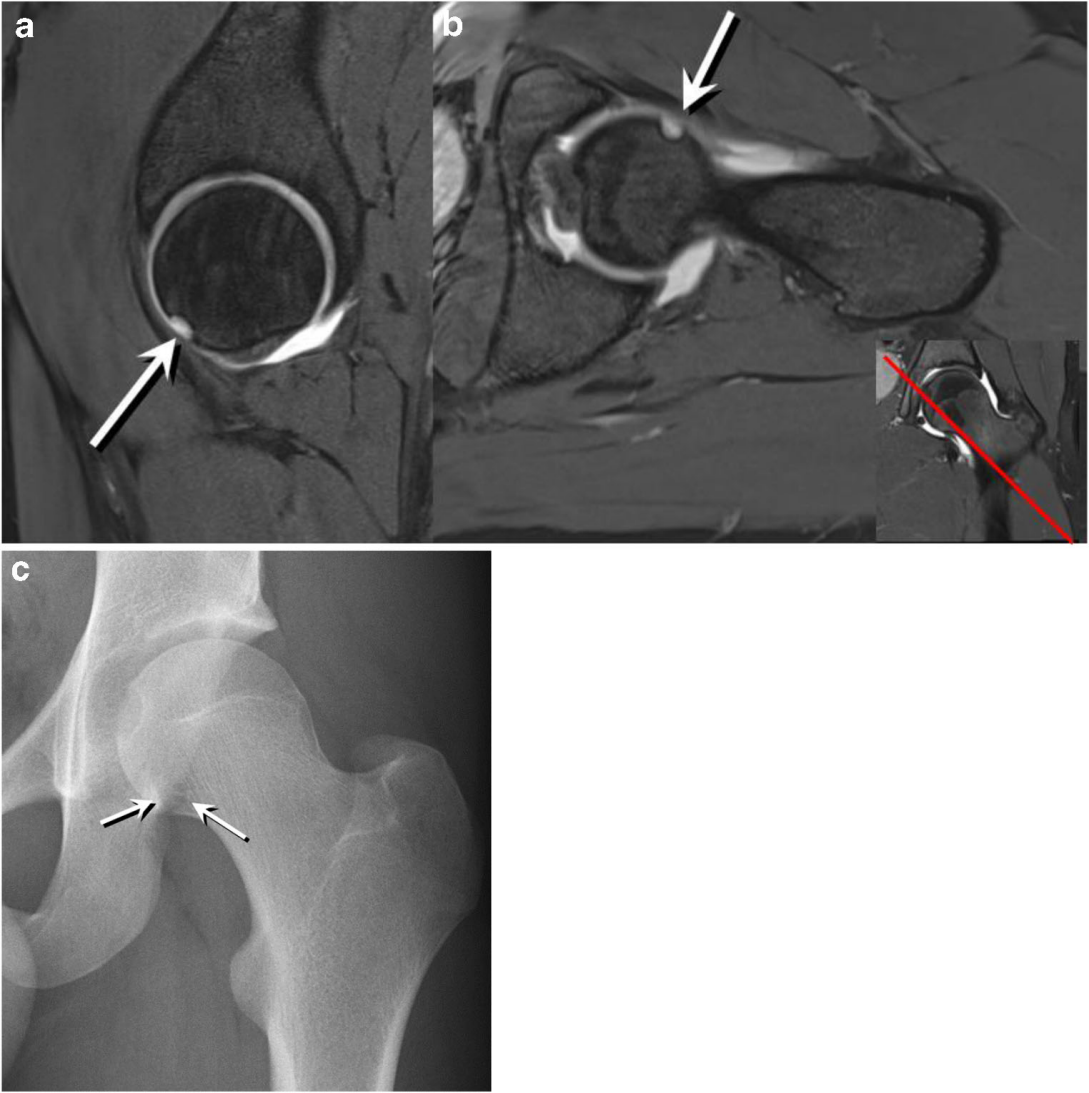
a Sagittal 3D double-echo steady-state MR arthrography image (25/9) of the left hip of an 18-year-old man. Osseous crater-like defect at the anteroinferior femoral head (arrow) corresponding to a type II FHD. b Transverse oblique three-dimensional water-excitation true fast imaging with steady-state precession MR arthrography image (repetition time msec/echo time msec, 12/6) of the left hip of the same patient. Type II FHD at the anteroinferior femoral head (arrow). Insert in b Coronal image with a red reference line indicating the location of the transverse oblique image, confirming the location of the FHD in an anteroinferior location. c AP pelvic radiograph of the same patient. Crater-like type II FHD at the anteroinferior femoral head (arrows)
